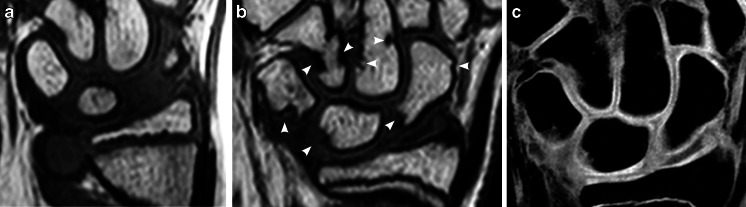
Carpal bones. a, b Coronal T1-weighted turbo spin-echo MRI in a 12-year-old boy (a) shows smooth carpal bones. Four-year follow-up MRI (b) shows several new bony depressions in almost all carpal bones (*arrowheads*). c Coronal water-selective (coronal volumetric steady-state spoiled gradient echo) cartilage sequence shows that all carpal depressions are covered by cartilage at follow-up
